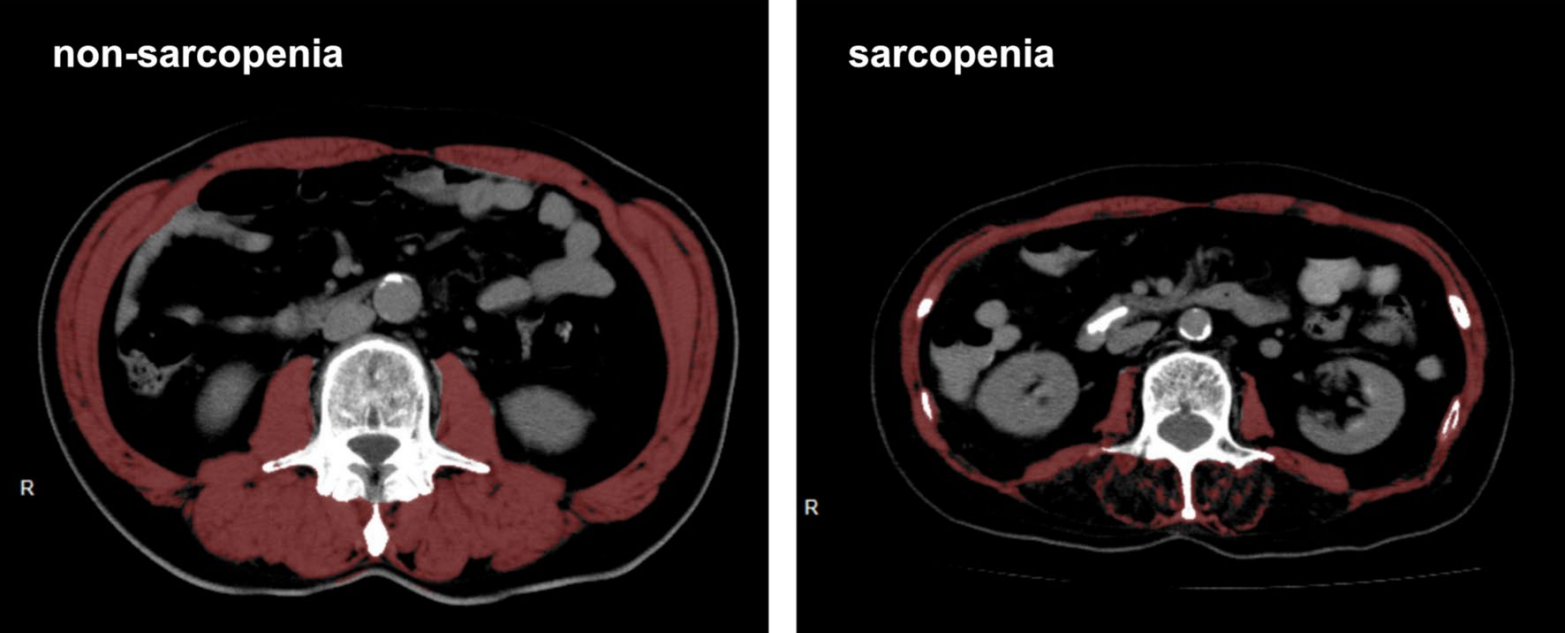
The cross-sectional computed tomographic images at the third lumbar vertebral body level in non-sarcopenic and sarcopenic patients; the red area indicates the total skeletal muscle area; skeletal muscle index (SMI) is the cross-sectional area of the total skeletal muscle/the square of the patient’s height (cm^2^/m^2^)
